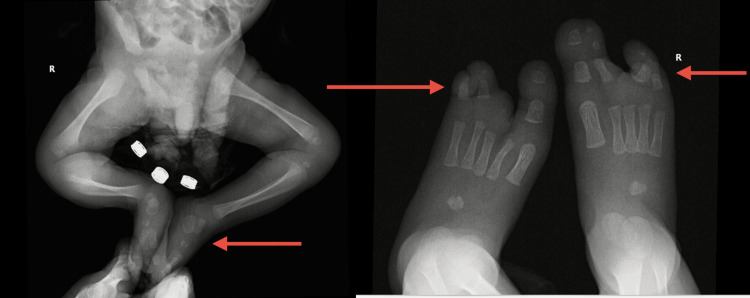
Anteroposterior radiograph of the lower limbs Images demonstrate bilateral talipes equinovarus with preserved hip joint alignment. Foot films reveal central ray deficiency consistent with ectrodactyly.
